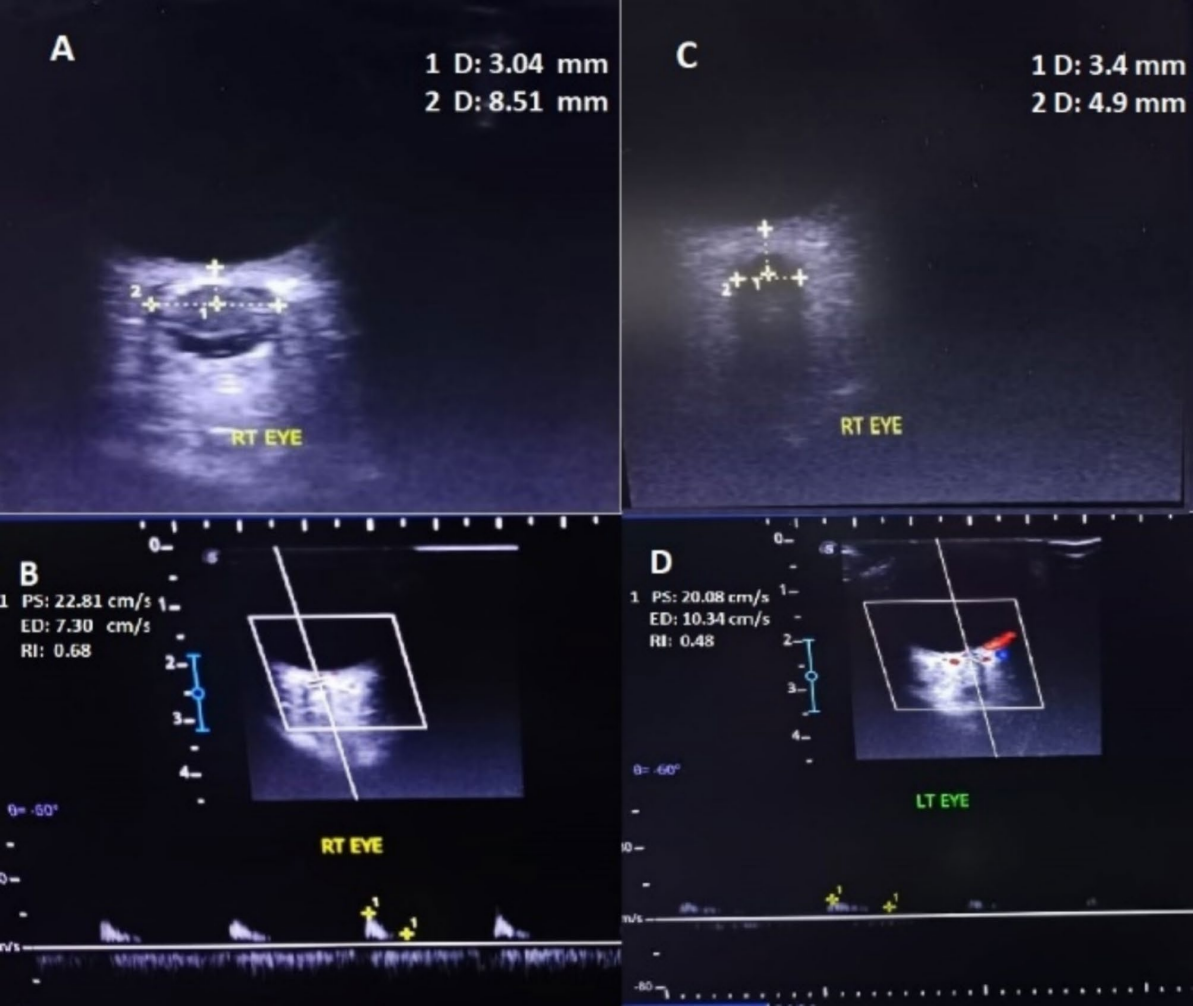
Trans-orbital Sonography in a patient with IH and control. (A, B) of IIH patient with papilledema reported increased ONSD = 8.5 mm with decreased EDV (7.3 cm/s) and increased RI (0.7) in comparison to normal control (C, D) who reported ONSD = 4.9 mm with EDV (10.3 cm/s) and RI (0.5).
